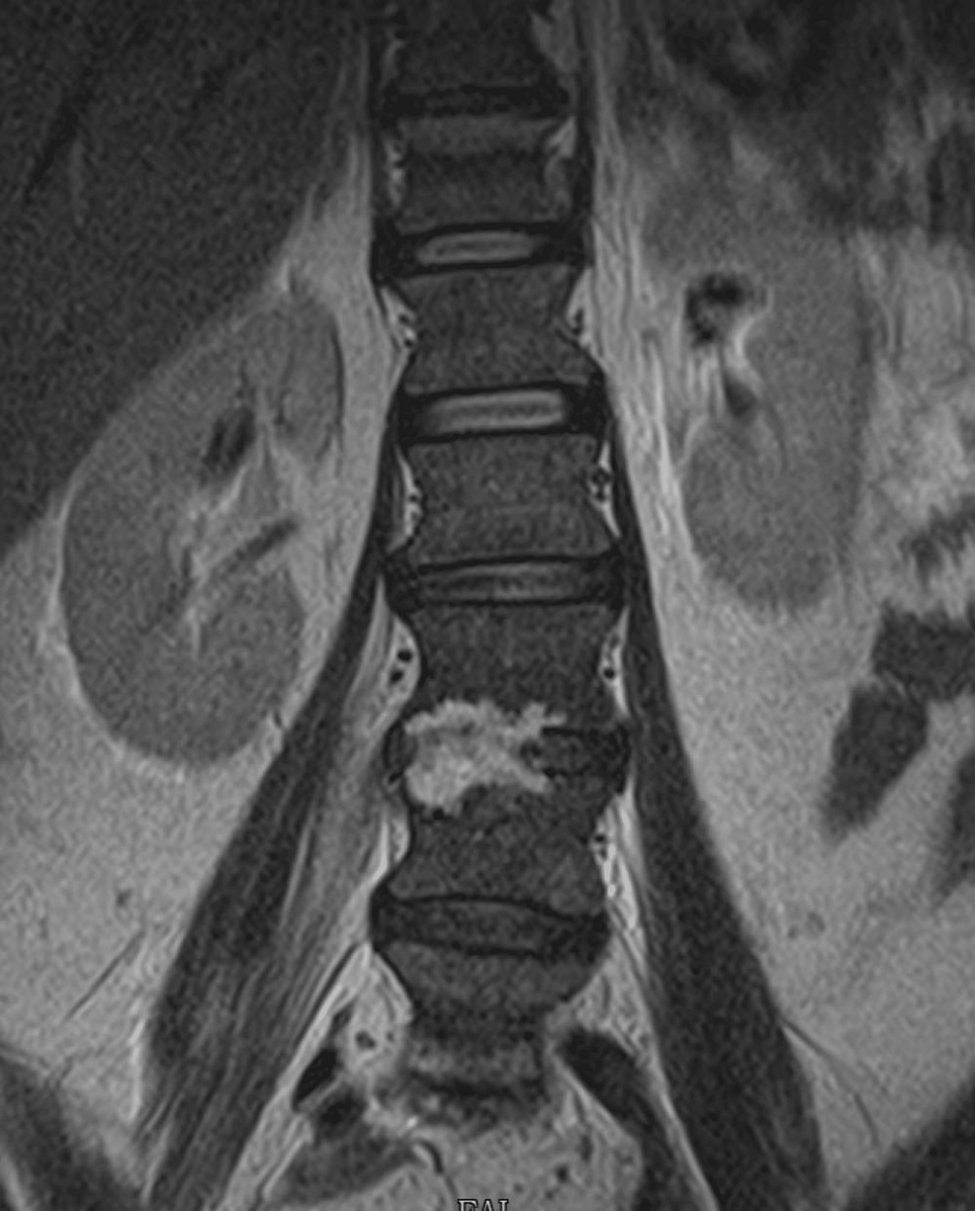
Magnetic resonance imaging (MRI) right before the beginning of the treatment (coronal view) Exuberant osteomyelitis is observed in the vertebral bodies of L3 and L4, but also in L5 and S1. Discitis is present between these vertebrae.
